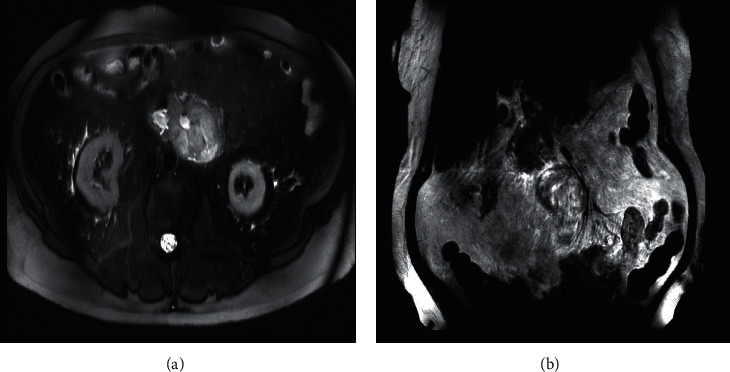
MRI abdomen confirming duodenal mass in both (a) sagittal and (b) coronal sections.
